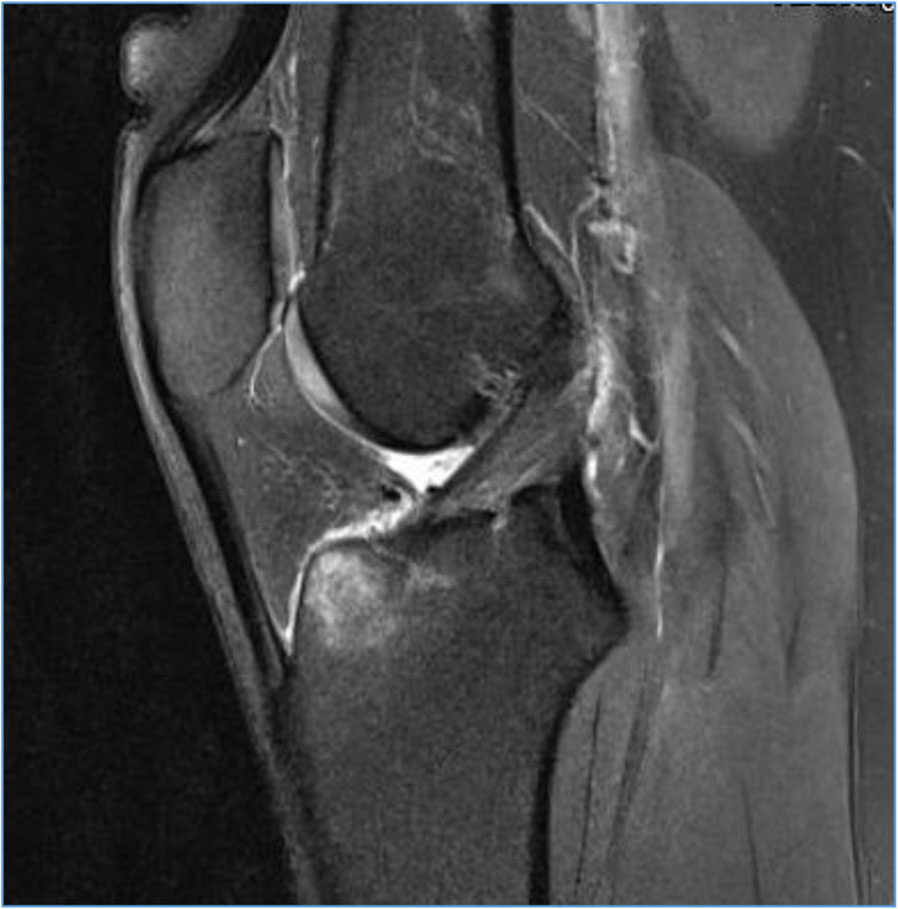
MRI scan of a 26-year-old male patient 2 years after primary arthroscopic suture anchor re-fixation of the ACL. The patient presented again in terms of a second injury including hyperextension of the knee. Up to this second injury, the patient was pain free and fully recovered to his preoperative sports level
